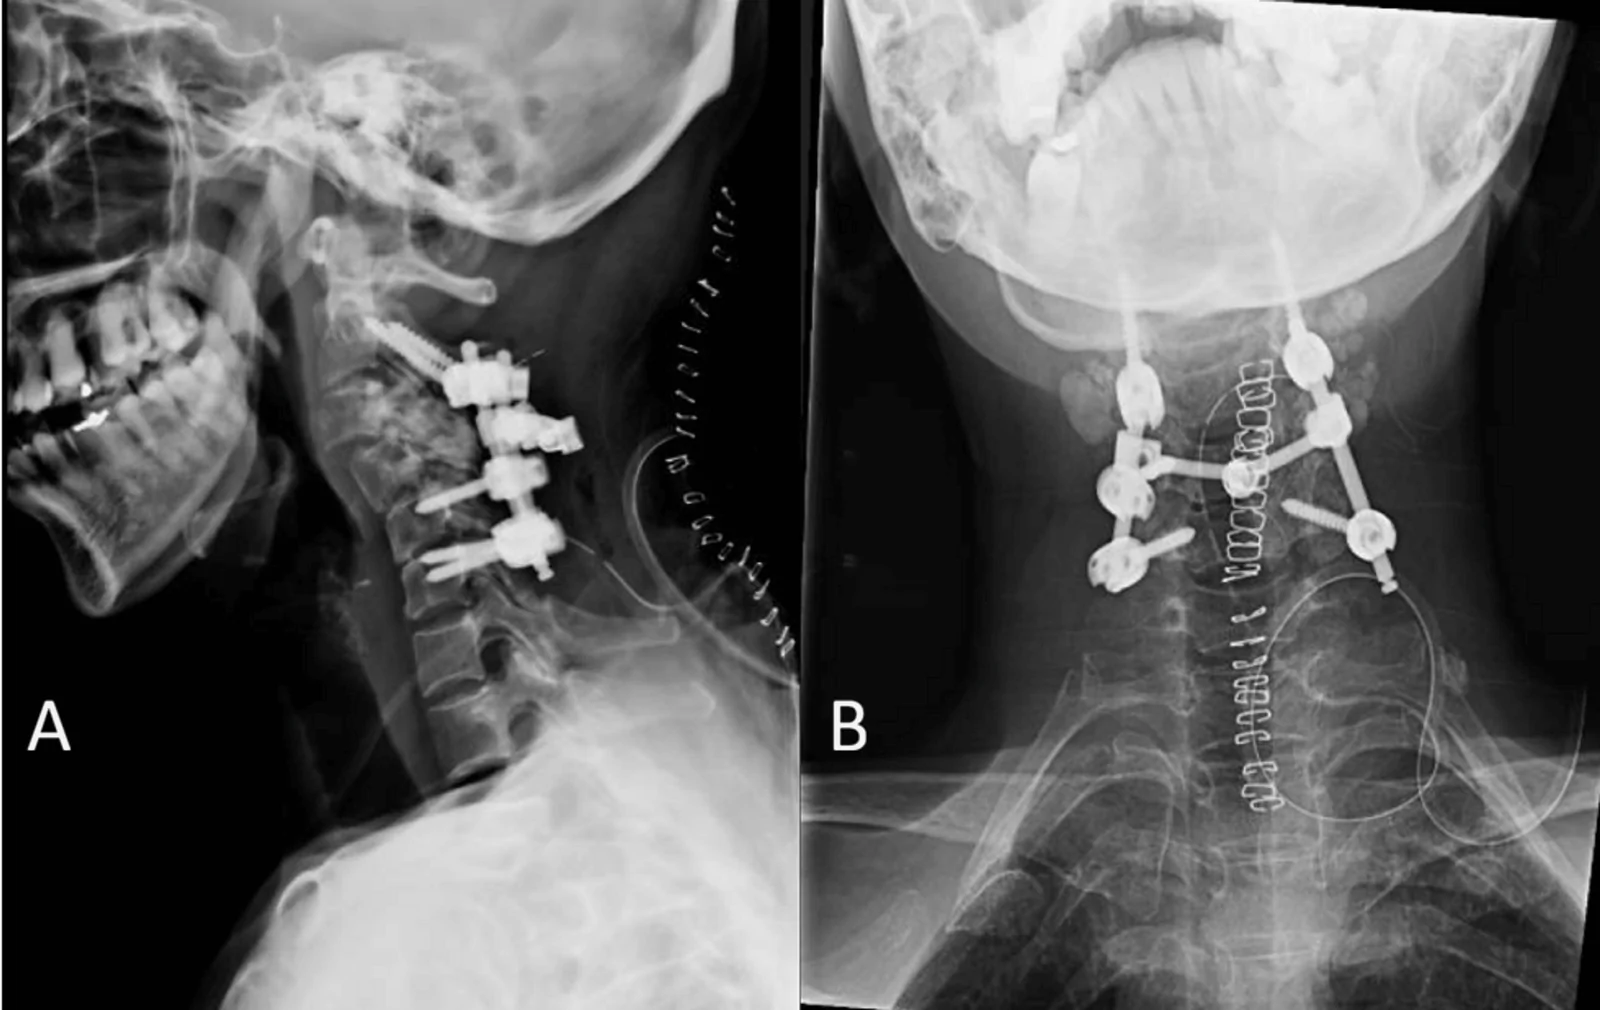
Cervical radiographs: (A) lateral and (B) anteroposterior views. Posterior cervical instrumentation consisted of bilateral C2 pars screws, a single right C5 screw, and bilateral C6 pedicle screws.
